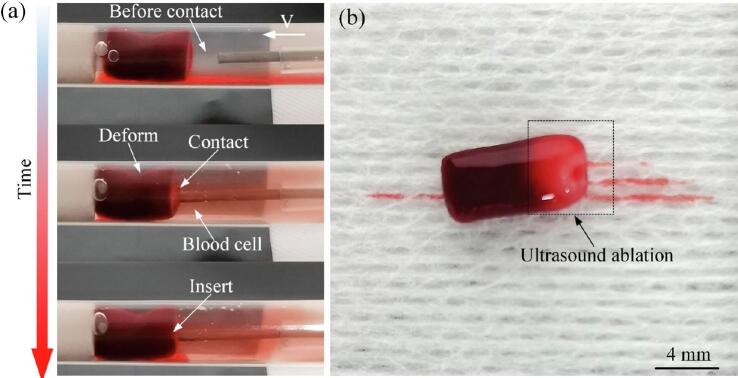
Ultrasonic ablation of thrombi. (a) Liquid turbidity changes. (b) Appearance of thrombus post-ablation.
